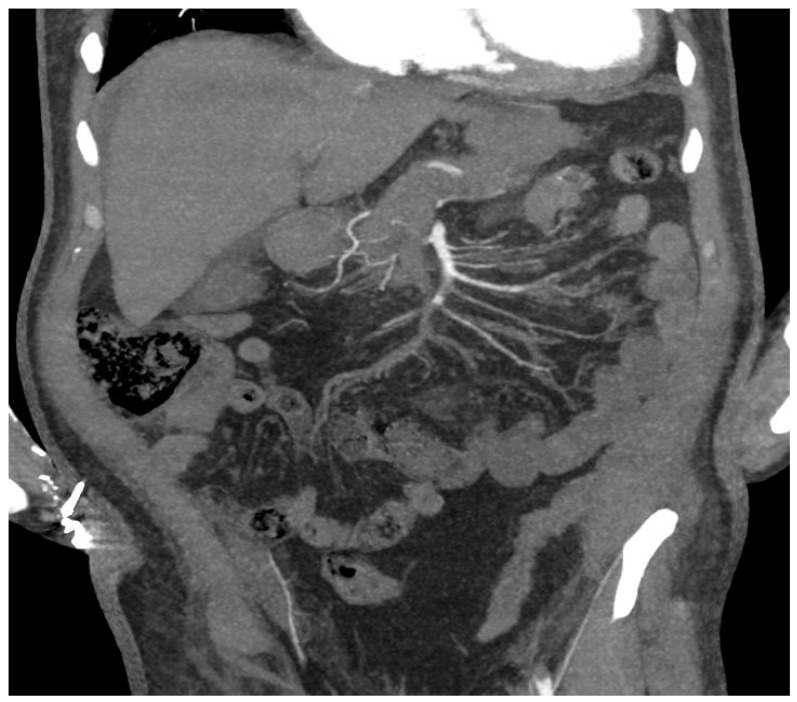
CT angiography, coronal planes, arterial phase, MIP reconstruction. Extended thrombosis in the superior mesenteric artery and its branches can be noted, with hypoperfusion of the intestinal loops.
